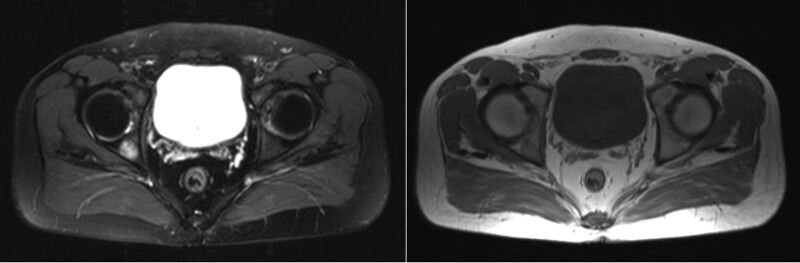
MRI scans of patient: (A) and (B) multiple bone and gluteal muscle metastasis. MRI = magnetic resonance imaging.
